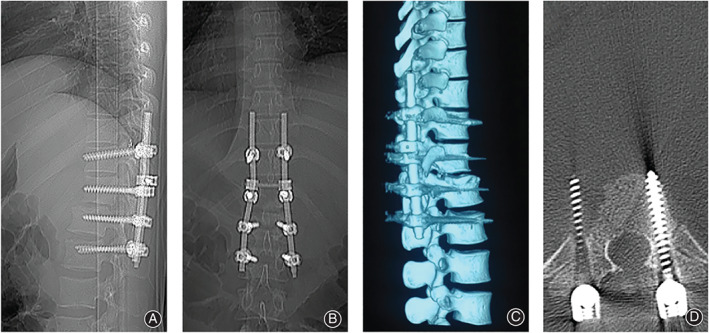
Plain radiographs after pedicle screw implant surgery. The dislocation of T_12_‐L_1_ was partly corrected (A). Both sides of the T_11_ and T_12_ pedicle screws seemed to have less TSA than the ones in the L_1_ and L_2_ vertebrae (B). Three‐dimensional reconstruction CT also showed the malplacement on the right side of the pedicle screw in L_2_ vertebra (C).Thoracolumbar CT shows malplacement of pedicle screws at T_12_ levels, which penetrated the aortic wall and formed a pseudoaneurysm of the aorta (D).
